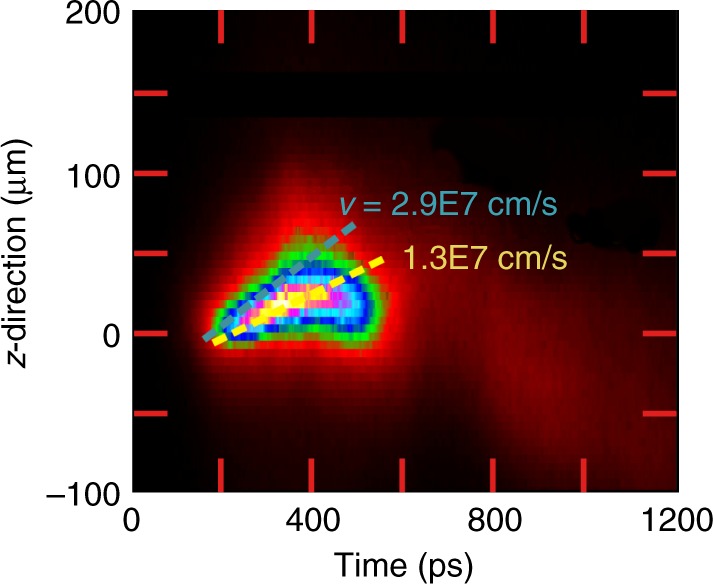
Plasma expansion visualized by X-ray streak camera. The laser is coming from below, the volume of the maximum emission moves with a velocity of $$1.3\times 1{0}^{7}\ {\rm{cm}}/{\rm{s}}$$
